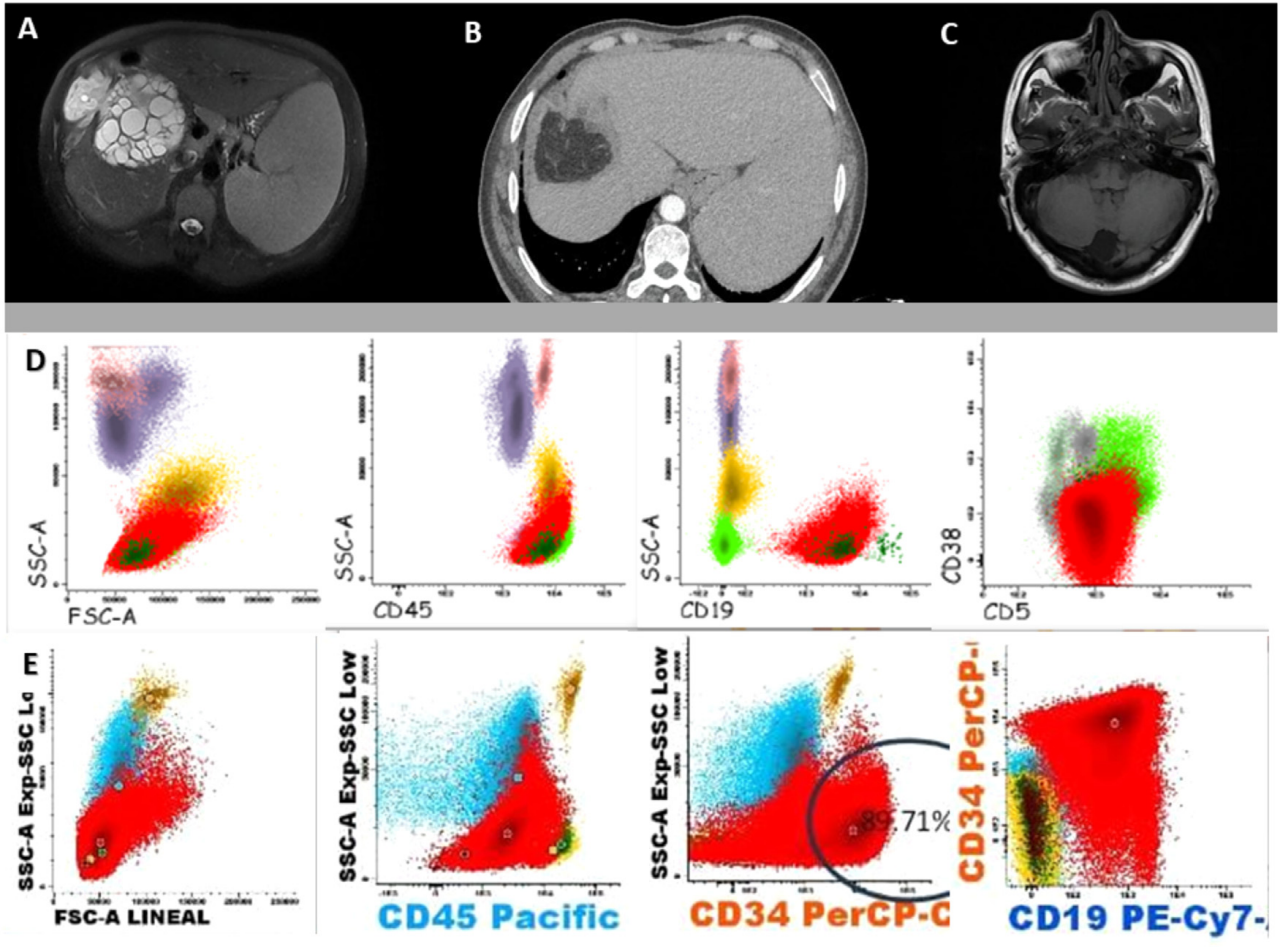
Imaging and immunophenotypic findings. (a) Abdominal magnetic resonance (August 24, 2022) imaging showing a large heterogeneous hepatic mass (129 × 163 × 143 mm) in the right lobe, with multiple daughter cysts and a peripheral hypointense capsule, findings consistent with a hepatic hydatid cyst (Case 1). (b) Abdominal CT (June 5, 2023) showing hepatomegaly with focal defect and absence of segment VIII, with an adjacent cystic image of ∼26 mm, consistent with postoperative changes. Splenomegaly is also observed (Case 1). (c) Axial T1-weighted spin echo brain magnetic resonance imaging without contrast showing a well-defined cystic lesion in the posterior fossa, consistent with an infratentorial arachnoid cyst, with mild displacement of the left cerebellar hemisphere (Case 2). (d) Flow cytometry of peripheral blood showing an aberrant mature B-cell population expressing CD19 and CD5, consistent with chronic lymphocytic leukemia (Case 1). (e) Flow cytometry of peripheral blood showing a blast population with low CD45 expression and positivity for CD34 and CD19, consistent with B-cell acute lymphoblastic leukemia (Case 2).
